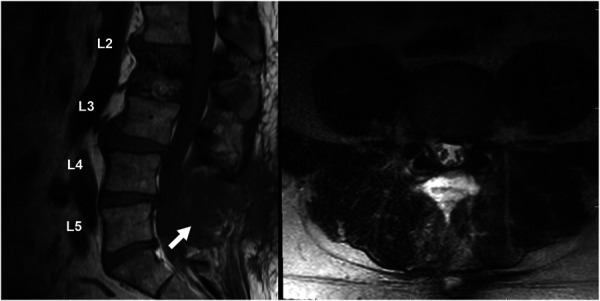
Postoperative MRI—Case 2. The sagittal T1-weighted (left) and axial T2-weighted (right) images demonstrate complete resolution of the posterior epidural lesion at L4-5 (white arrow, left) and confirm the decompression of the spinal canal with no residual mass.
